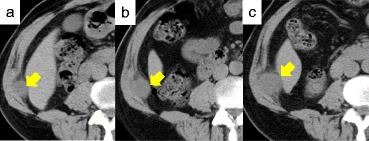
Computed tomography image of the right mass in a) October 2014, b) October 2015, and c) October 2016 (arrow; tumor)
